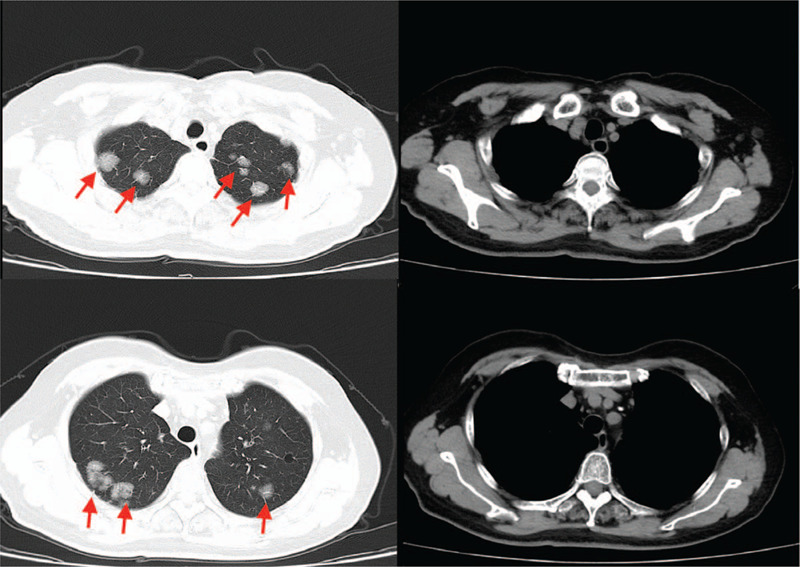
After 1 month of onset, chest CT shows multiple grinding nodes in both lungs. CT = computed tomography.
